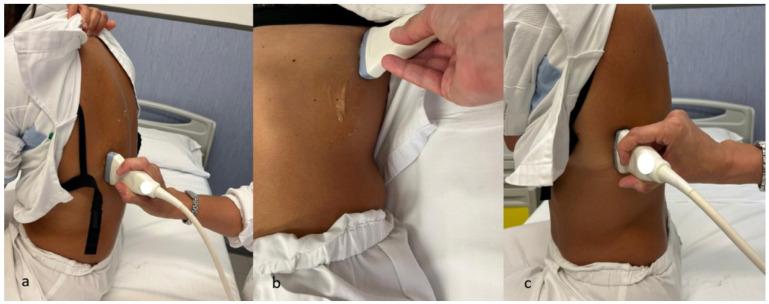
Probe positioning for ultrasound assessment of the thoracic wall: (a) Posterior thoracic scan: the patient is seated, and a linear probe is placed perpendicular to the ribs in the posterior thoracic region. (b) Anterior thoracic scan: with the patient in the supine position, the linear probe is applied perpendicular to the ribs on the anterior chest wall. (c) Lateral thoracic scan: the patient is seated, and the probe is positioned perpendicular to the ribs in the lateral thoracic area.
